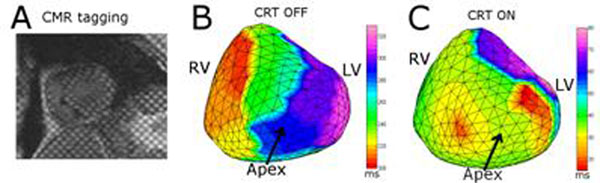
(A)Mid-myocardial, mid-systole short axis MRI tagging image in a patient with CRT device showing sufficient tag quality for strain analysis. (B)Reconstructed electrical activation times during intrinsic activation showing RV preceding LV activation. (C)Synchronized electrical activation of the same patient during biventricular pacing.
